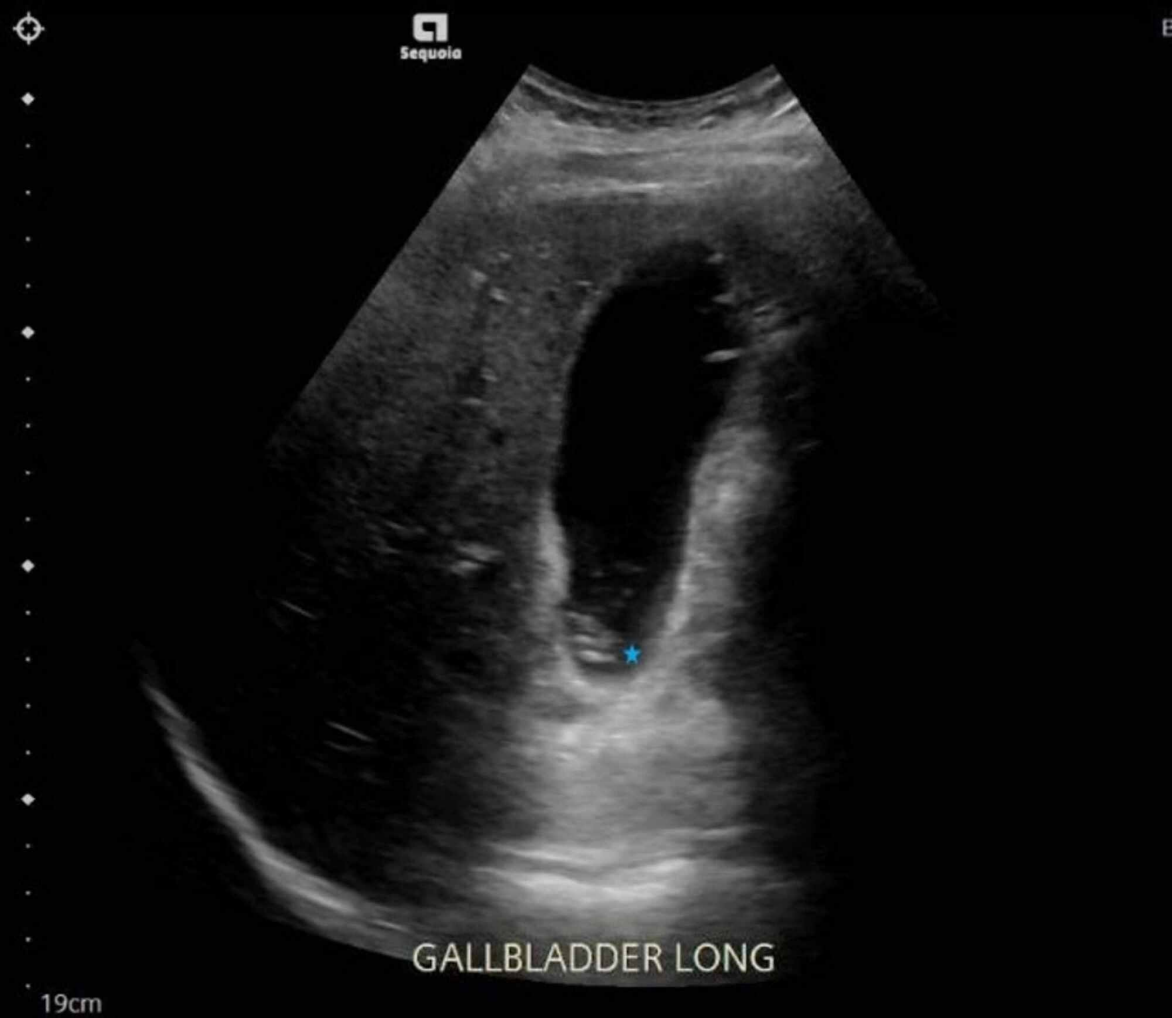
Ultrasound of the abdomen demonstrating gallstones (star) without evidence of acute cholecystitis
